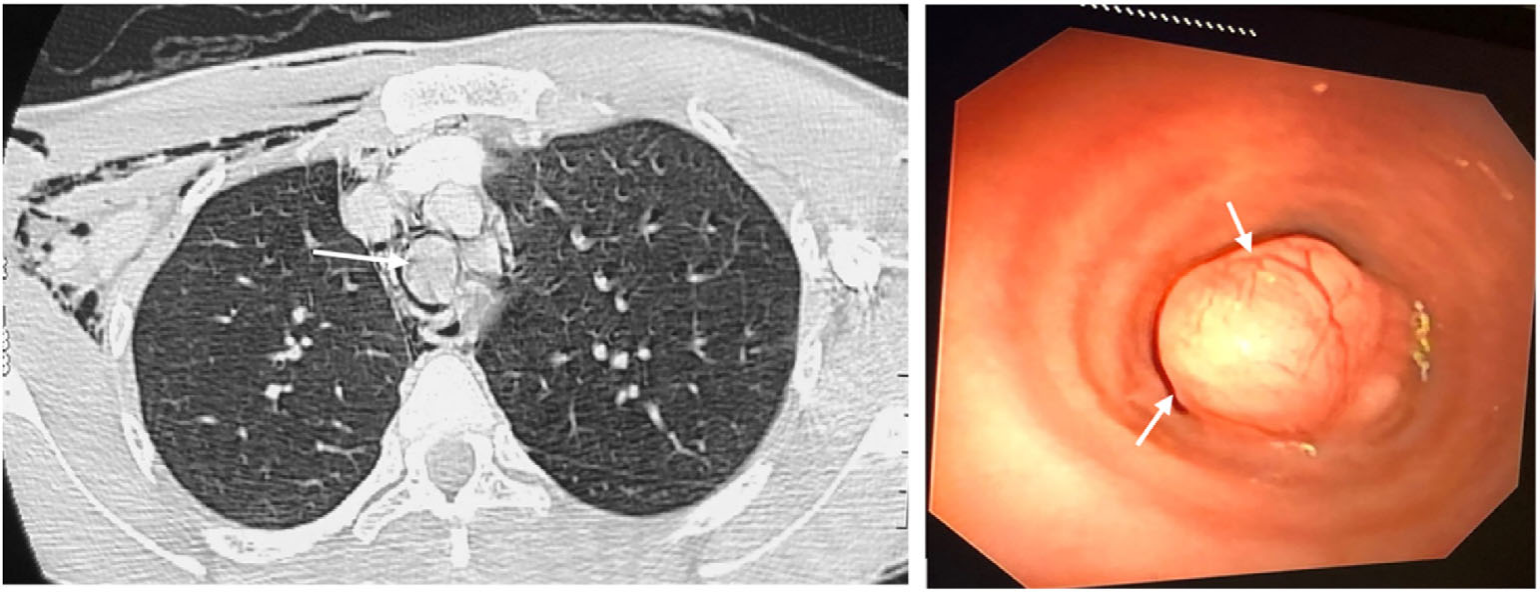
(A) Chest CT scan lung window image depicting the occluding tracheal mass (*long white arrow*) and right chest wall/ axillary soft tissue emphysema. (B) Bronchoscopy image demonstrating a smooth surfaced tracheal mass (*short white arrow*) causing severe airway stenosis.
